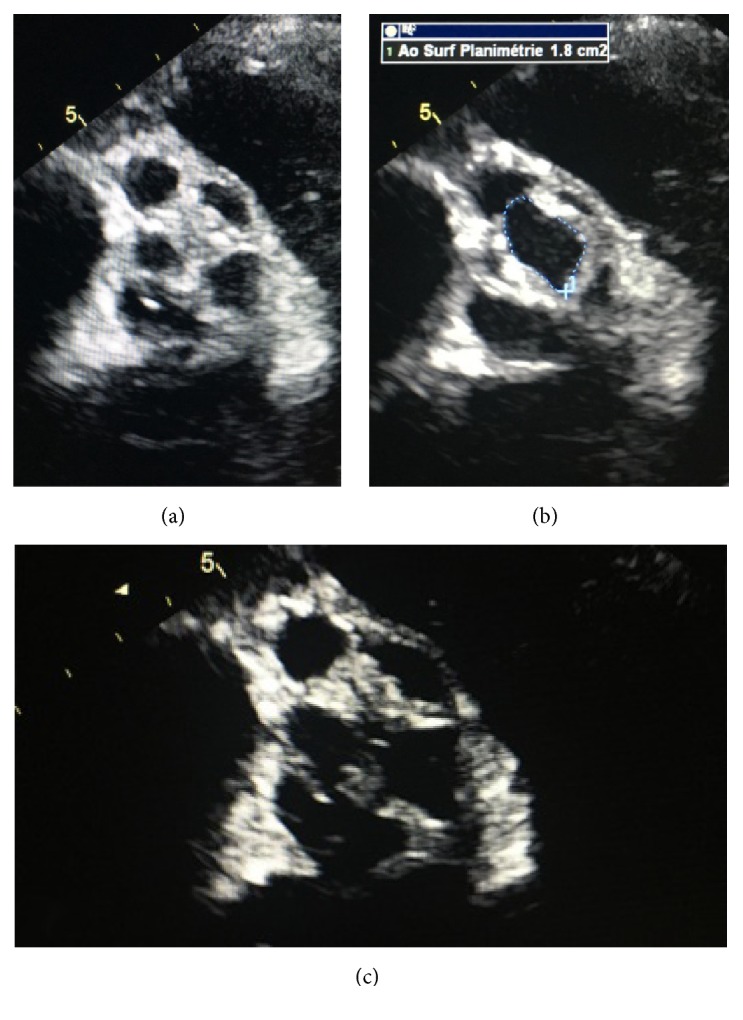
TTE: parasternal short axis view showing type A quadricuspid aortic valve in systole (a) and diastole with a planimetric aortic valve area of 1.8 cm^2^ (b). (c) shows type A quadricuspid aortic valve during systole along with two fistulae, the first between the left sinus of Valsalva and the left atrium (LA) and the second between an aortic periannular abscess and LA.
